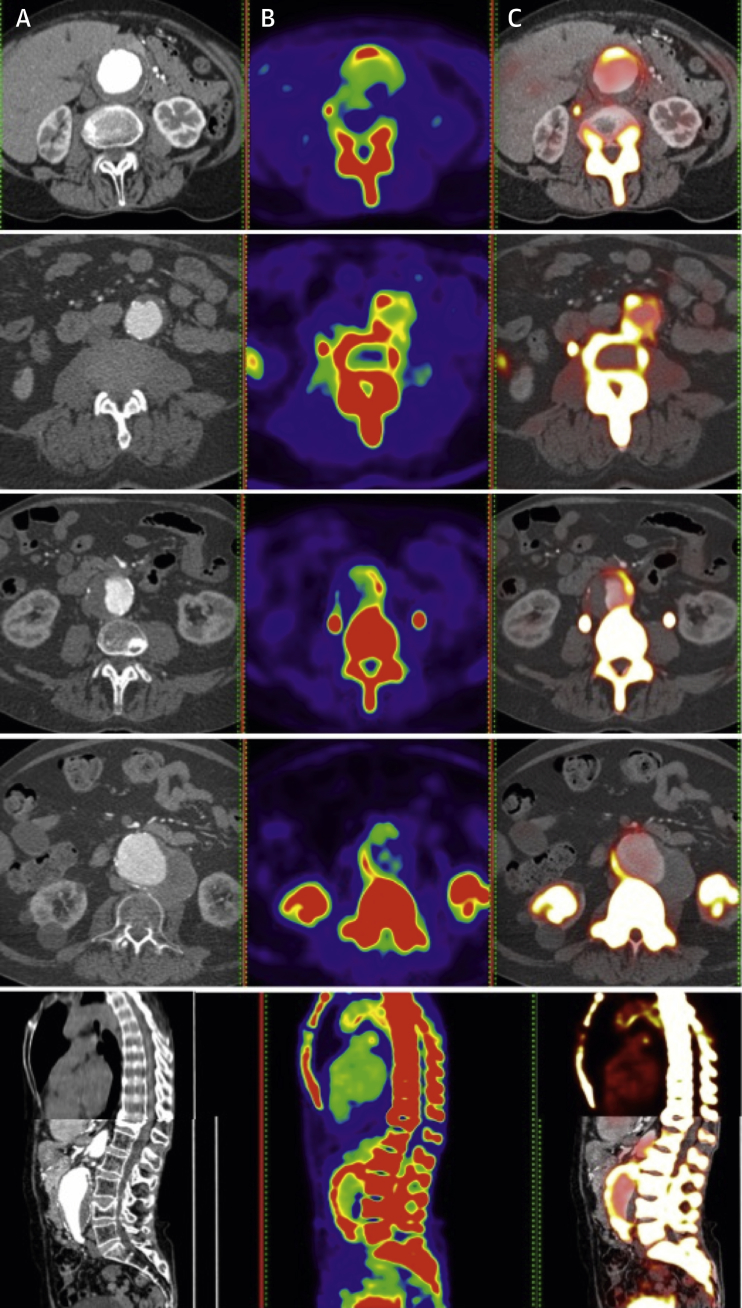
Positron Emission Tomographic and Computed Tomographic Images of Abdominal Aortic Aneurysms (A) Structural image of computed tomographic angiography, (B)^18^F–sodium fluoride uptake on positron emission tomography, and (C) fused positron emission tomographic–computed tomographic images colocalizing ^18^F–sodium fluoride uptake with the skeleton and abdominal aortic aneurysm.
